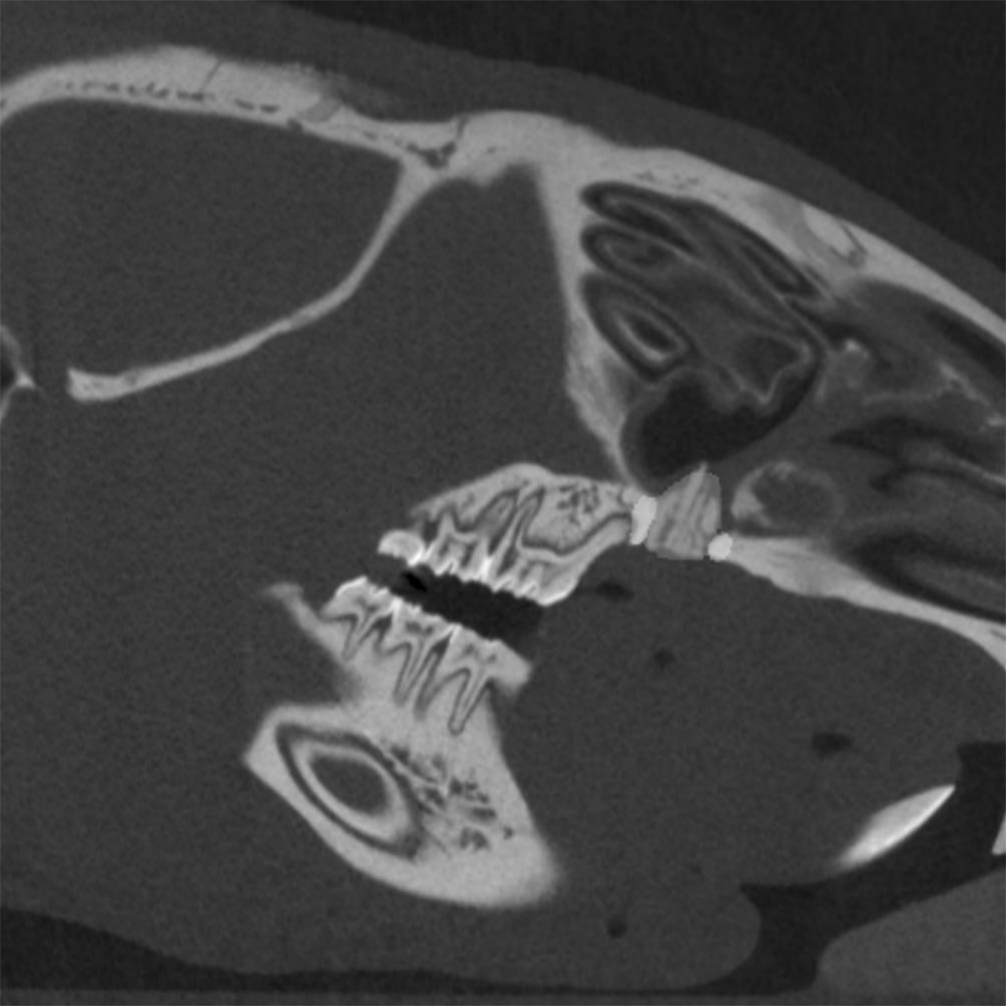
Sagittal view of a CT scan after cleft repair for analyzing the bone quality of the augmented bone into the cleft (dark grey/green area) and the surrounding alveolar bone (light grey/beige area).
